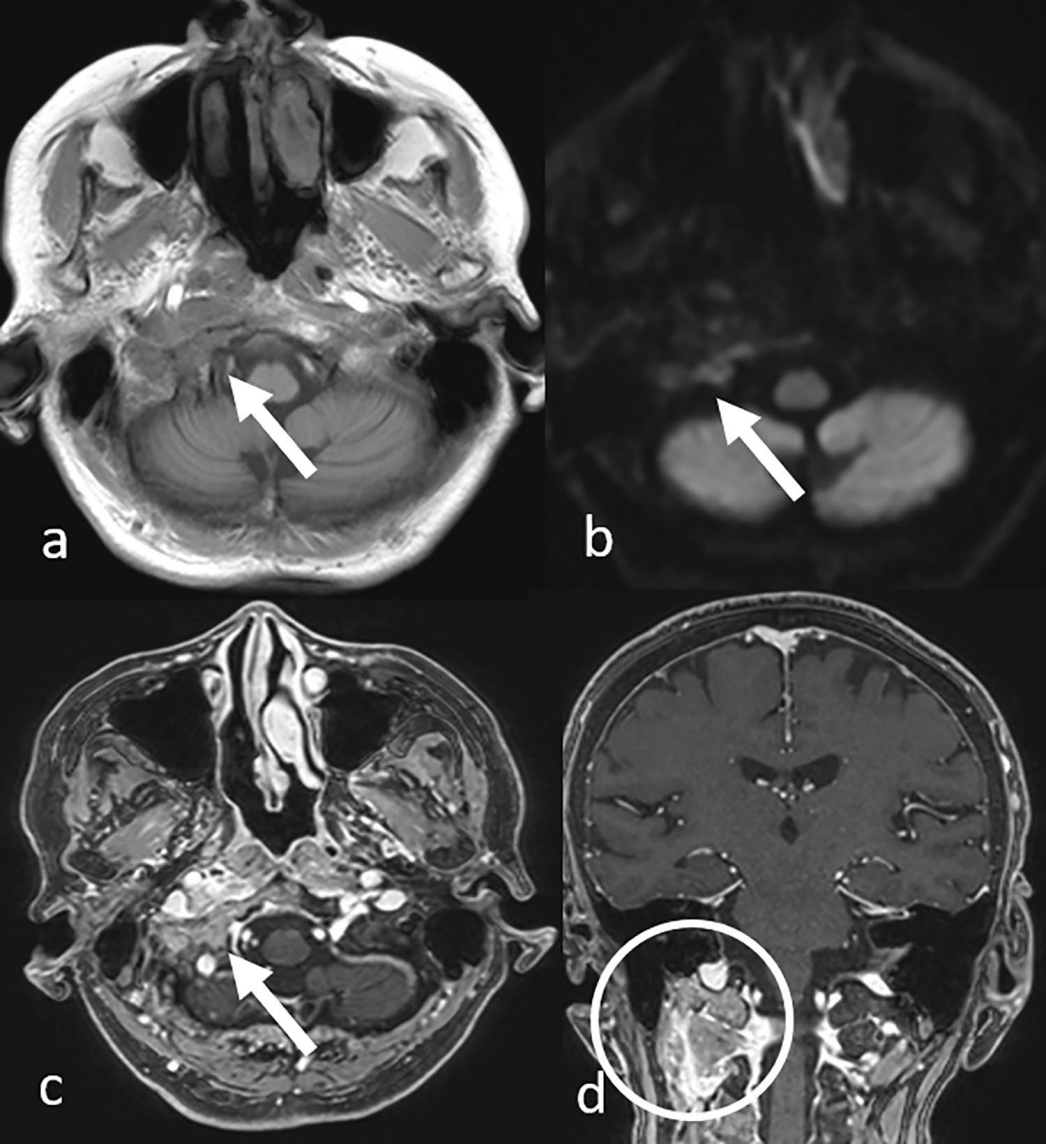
Osteolytic skull base metastasis in a patient with lung adenocarcinoma. T1-weighted a and diffusion-weighted b images show part of the tumor (arrows). Contrast-enhanced 3D fat-suppressed T1-weighted images with multiplanar reconstruction in the axial c and coronal d views show the tumor invading extracranial structures (c, arrow and d, circle)
